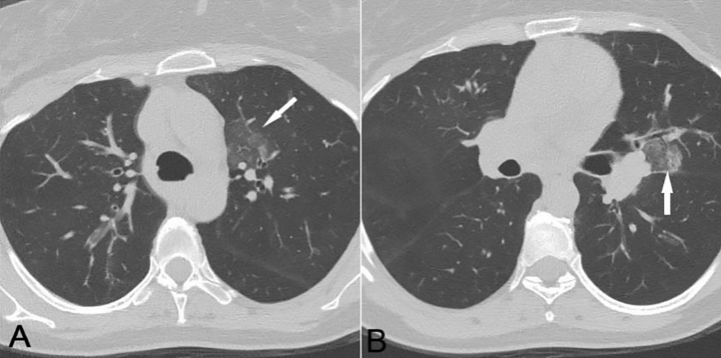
Axial chest CT images of a known case of Churg-Strauss demonstrate patchy transient nonsegmental opacities with no zonal predilection. Air way involvement such as bronchial wall thickening and bronchiectasis may also be detectable.
